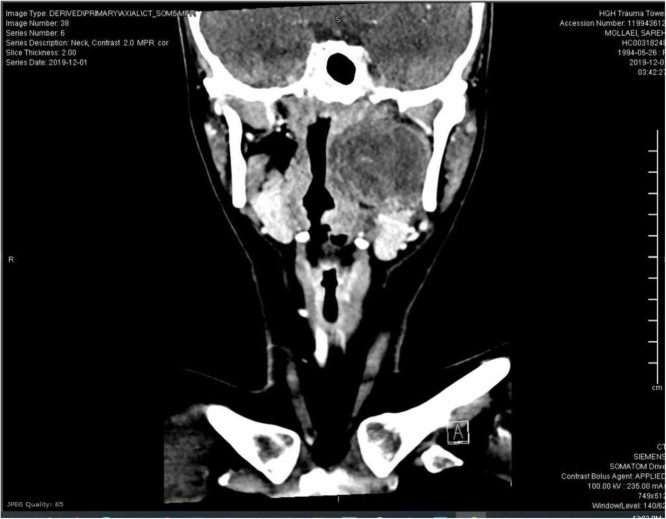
Coronal Cut of Neck CT with contrast.
